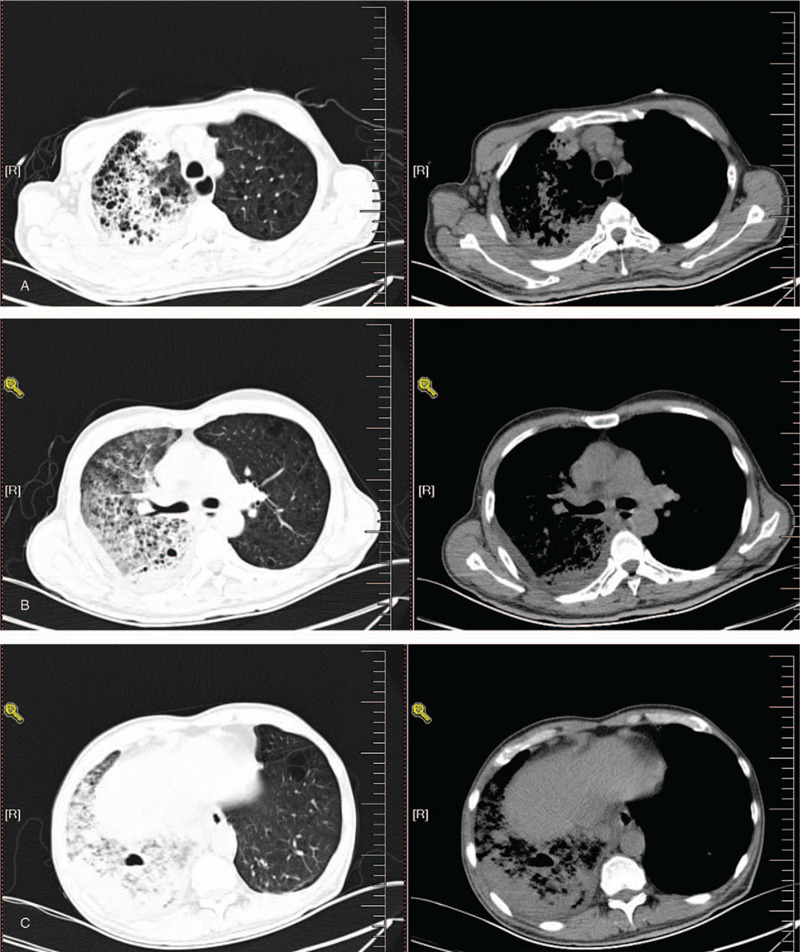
A–C, Pulmonary CT upon admission. Diffuse ground-glass opacity of the right lung with lower lobe consolidation; right lower lobe cavities with fluid level; right pleural effusion.
